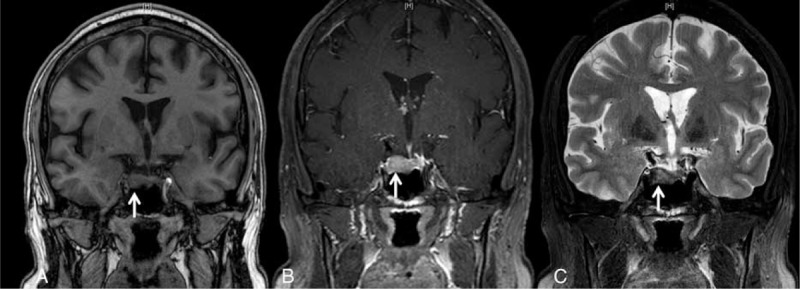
Pituitary magnetic resonance imaging. T1-weighted coronal (A), gadolinium-enhanced T1-weighted coronal (B), and T2-weighted coronal (C) views showing a 15 mm macroadenoma.
